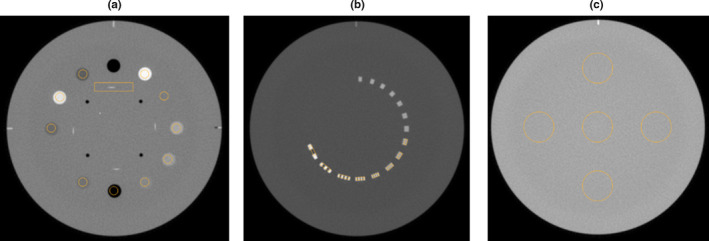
Registered cone‐beam computed tomography scan of the Catphan® 604 in SunCHECK Machine. Catphan modules shown in (a), (b), and (c) are used for analysis corresponding to parameters presented in Table 3.
